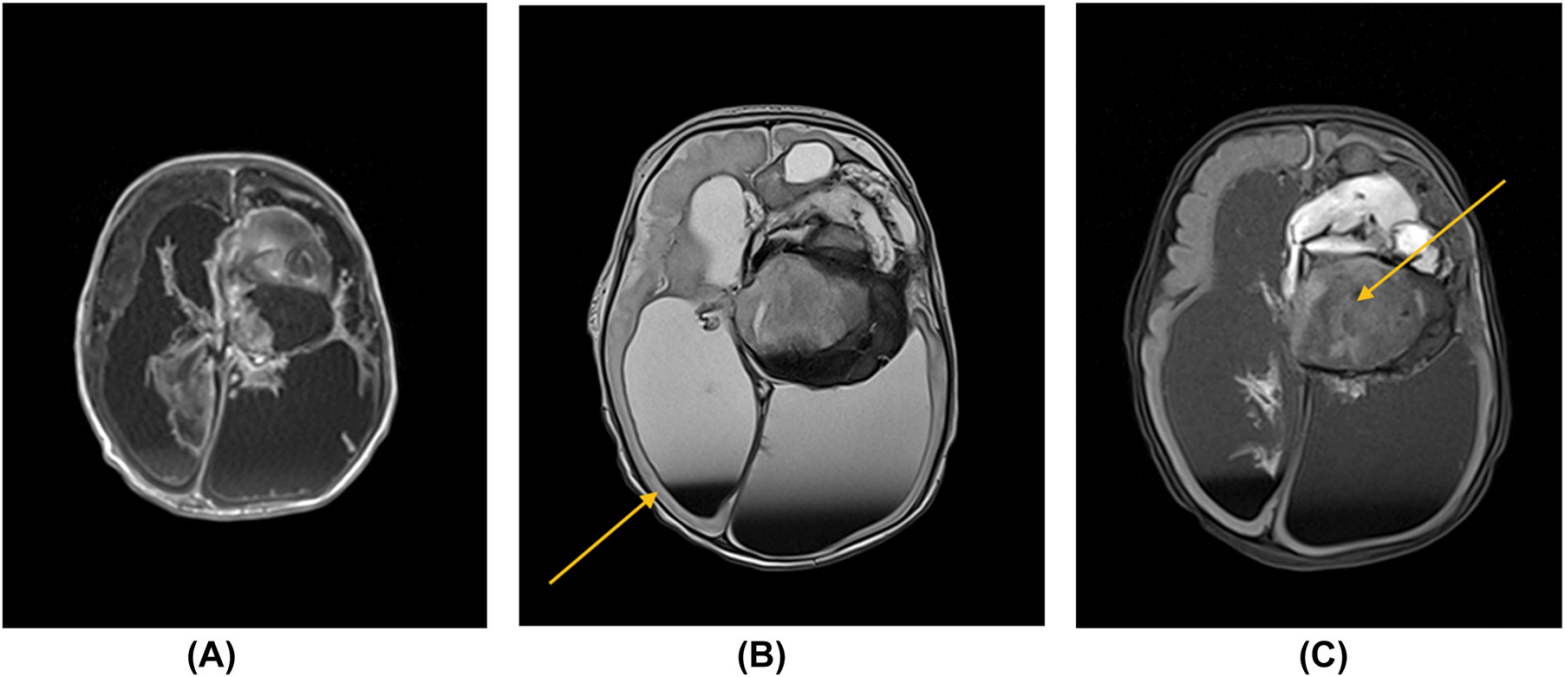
Newborn head MRI at 2nd day of life (A–C). In the image B an arrow marks the brain tissue. The tumor mass is marked with an arrow in image C.
